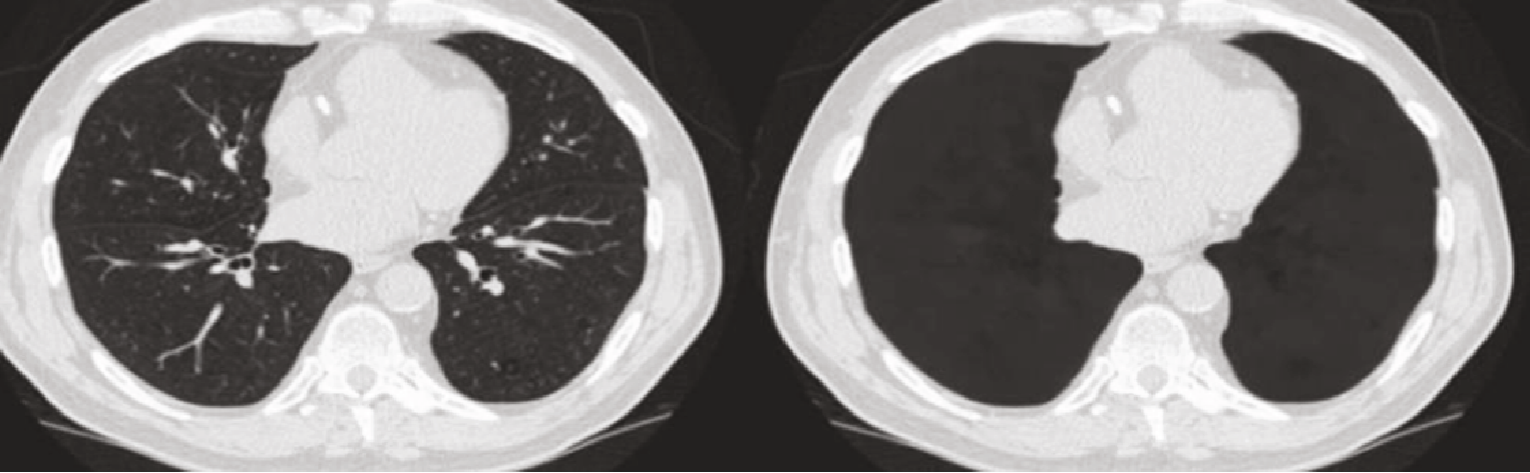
Contrasted enhanced CT thorax postintervention noted resolution of pleural effusion and empyema.
